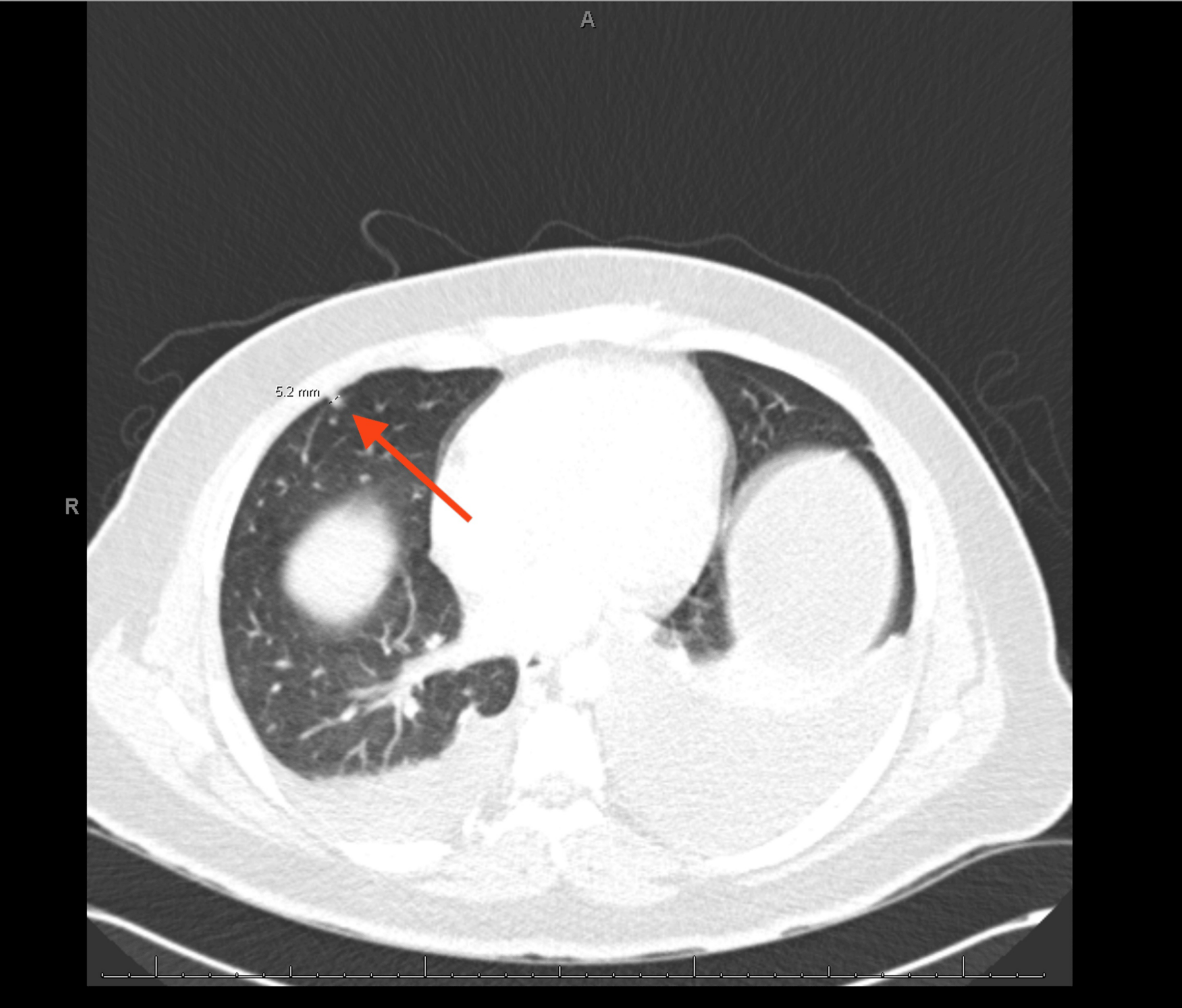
CT scan showing a 5 mm pulmonary nodule. The red arrow indicates a 5 mm pulmonary nodule in the right lung.
